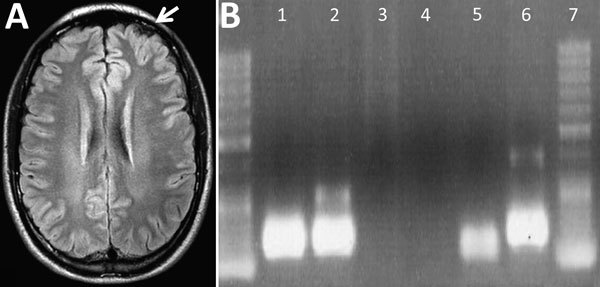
Imaging and PCR results for a 28-year-old man with Oropouche virus infection, southeastern Brazil. A) Cerebral computed tomography showing a cortical edema on the left frontal lobe (white arrow). B) Agarose gel of reverse transcription PCR products of Oropouche virus S fraction. Lane 1, patient’s serum sample; lane 2, patient’s cerebrospinal fluid; lane 3, patient’s leukocyte supernatant; lane 4, negative control; lane 5, internal control; lane 6, positive control.
